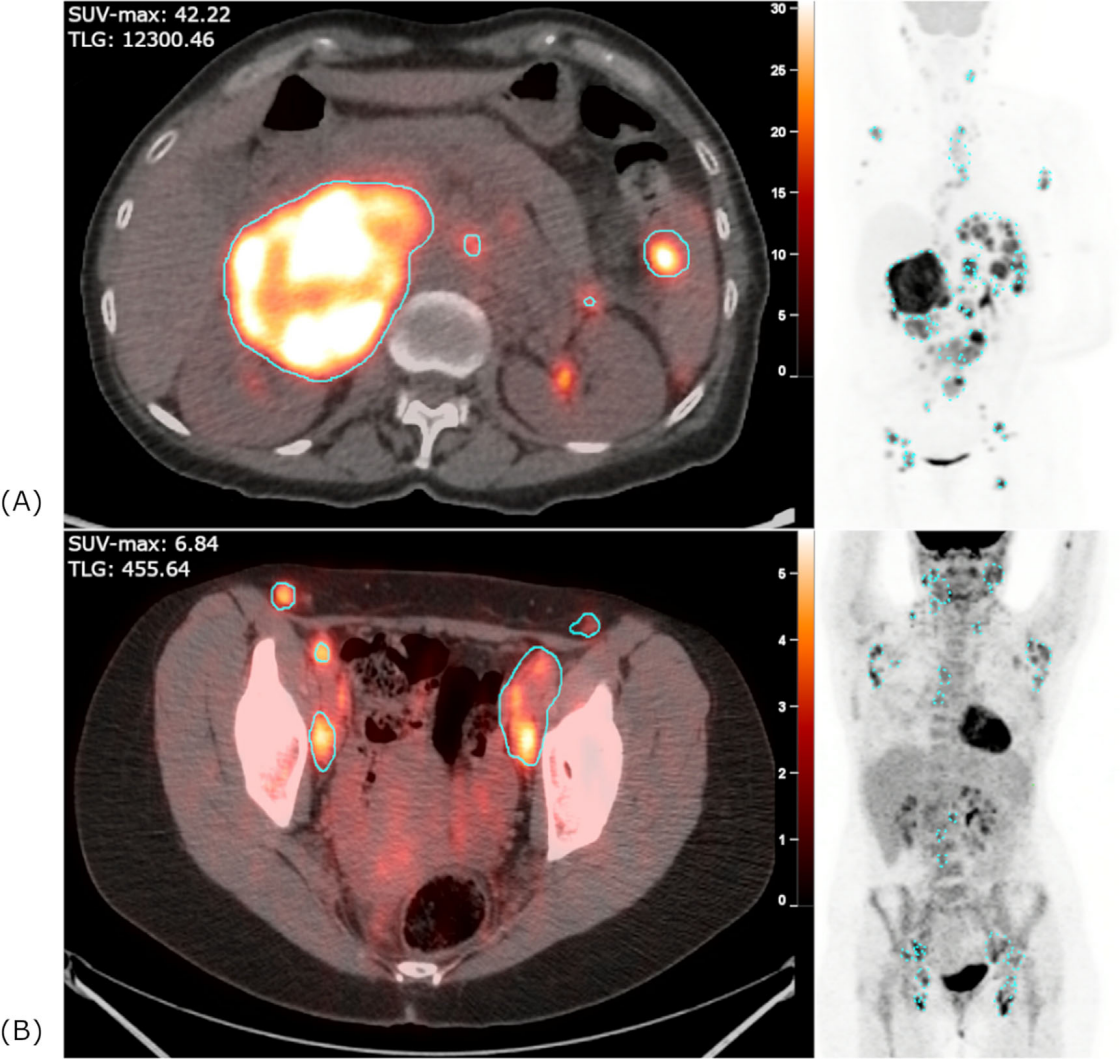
Representative example of a patient who experienced large cell transformation with high maximum standard uptake value (SUV_max_
